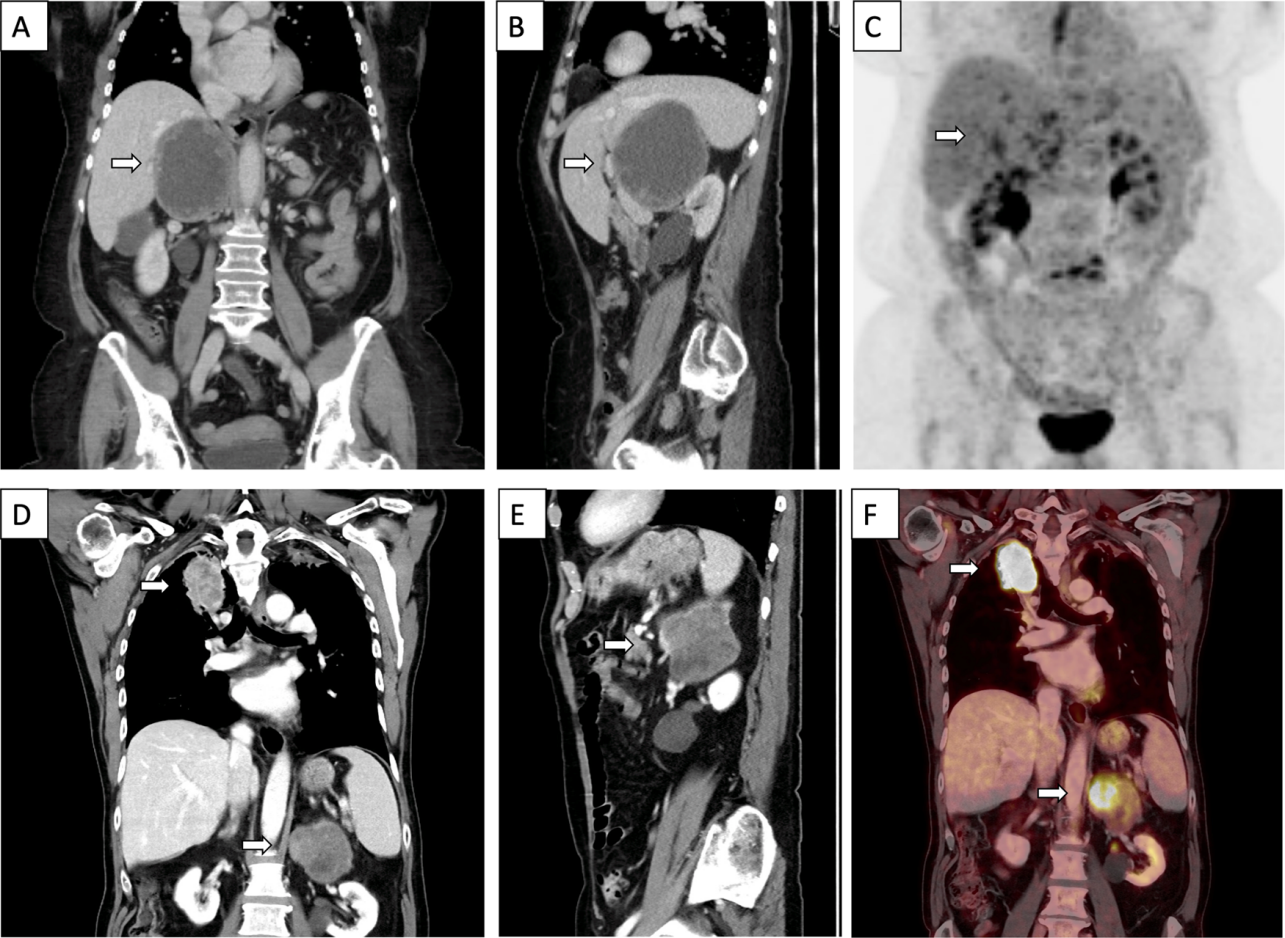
Radiological images of adrenal and periadrenal schwannoma. Depicted here are images of case 2 (A–C) and 4 (D–F). White arrows indicate the tumors in all images. A Coronal view of computerized tomography (CT) scan, showing the incidentally found 9 cm lesion cranial to the right kidney, suspected of originating from the right adrenal gland. B Sagittal view of the same CT scan as in A. C Coronal view of flourine-18 fluorodeoxyglucose positron emission tomography/computed tomography (^18^F-FDG PET/CT) containing the suspected adrenal mass with an assumed necrotic center and viable periphery. D Coronal view of CT scan showing both the 50 × 50 mm mass in the right upper lobe of the lung, and the 75 × 50 mm lesion in the left adrenal. E Sagittal view of the adrenal lesion described in D. F Coronal view of ^18^F-FDG PET/CT showing ^18^F-FDG uptake in both the pulmonary mass and in the left adrenal tumor
